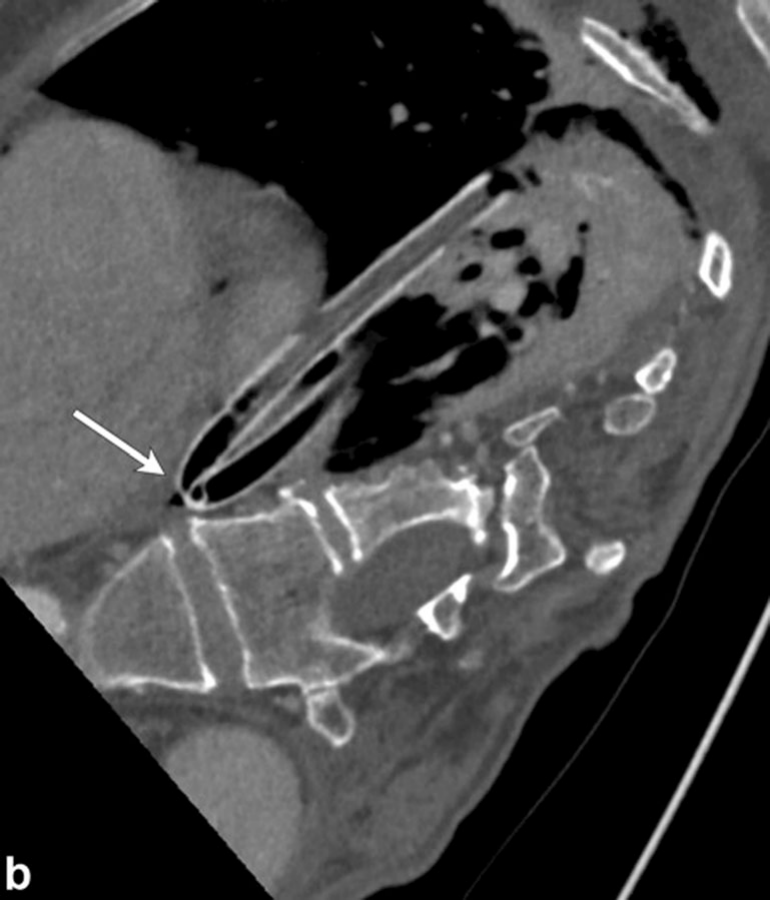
An 87-year-old female presenting with multiple rib fractures after falling down stairs. Frontal chest radiograph (a) and oblique multiplanar reconstruction from CT (b) demonstrate acute kink involving the chest tube (*arrow*); this was the etiology of a nonresolving pneumothorax
